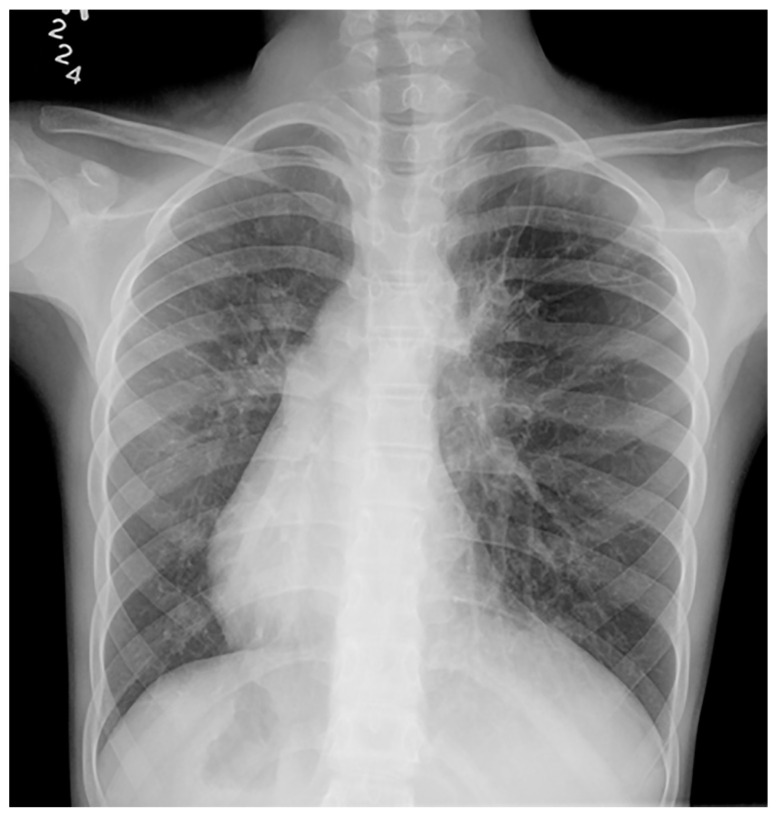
Frontal chest radiography in a young patient with confirmed Kartagener syndrome shows the characteristic complete situs inversus with dextrocardia and stomach on the right, and liver on the left within the abdomen. Bronchiectasis is noted in the left lung.
